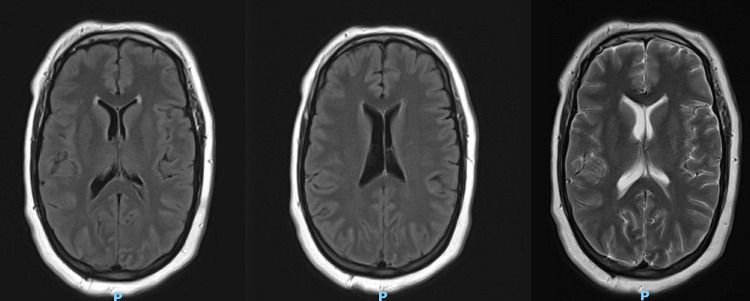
Normal brain MRI with and without contrast. MRI: magnetic resonance imaging
